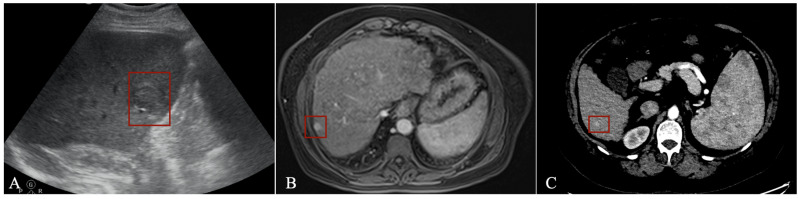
Detection algorithm using ultrasound (A), MRI (B) and CT (C) imaging in the arterial phase.
